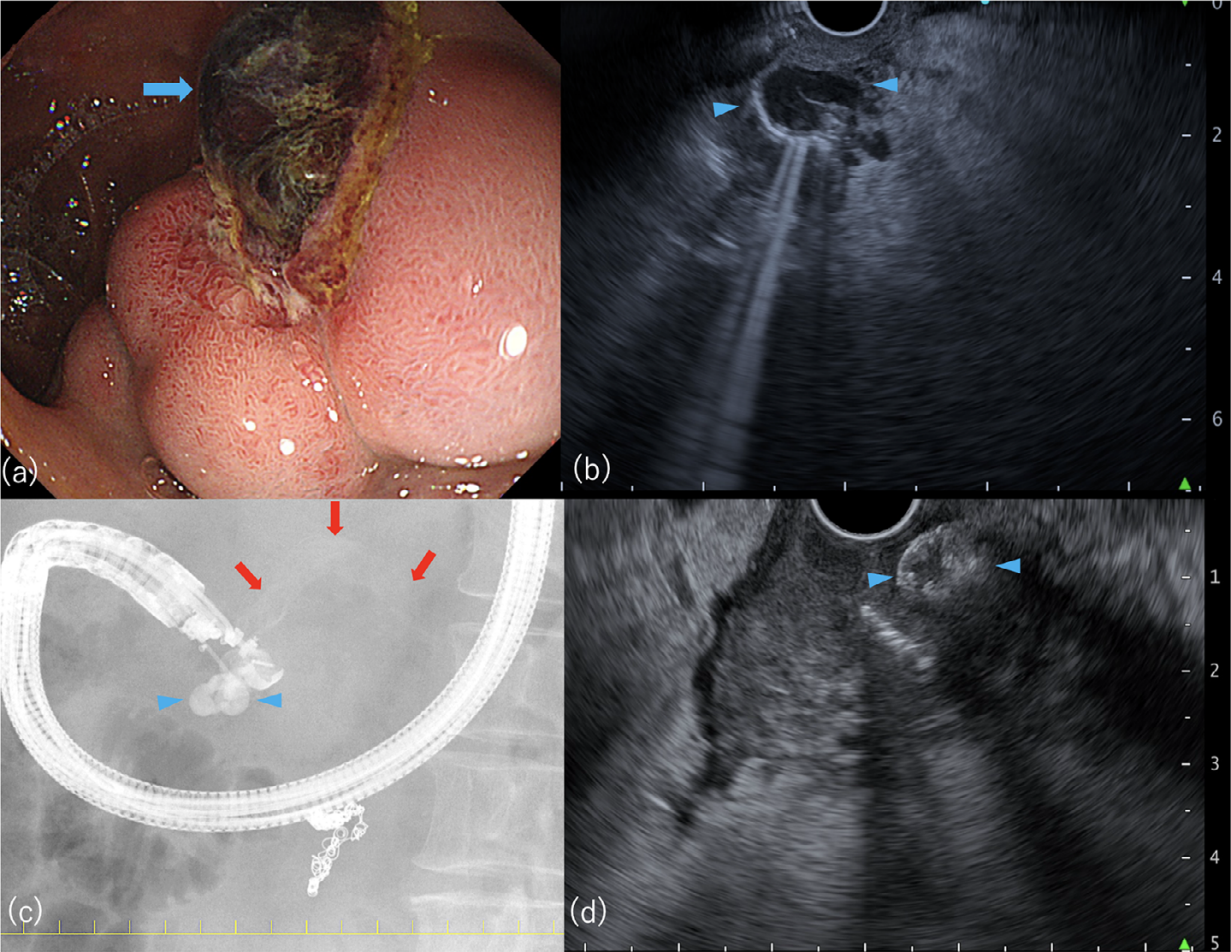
Endoscopic and endoscopic ultrasonography (EUS) images at the time of rebleeding. (a) Rupture site identified using endoscopy. (b) Duodenal varix visualized on EUS (short axis, 5 mm). (c) N‐butyl cyanoacrylate (NBCA) injected during EUS‐guided variceal therapy (EUS‐VT). (d) Injected NBCA visualized on EUS. Blue arrow: rupture site of the duodenal varix. Blue arrowhead: duodenal varix. Red arrow: feeder vein originating from the distal superior mesenteric vein.
